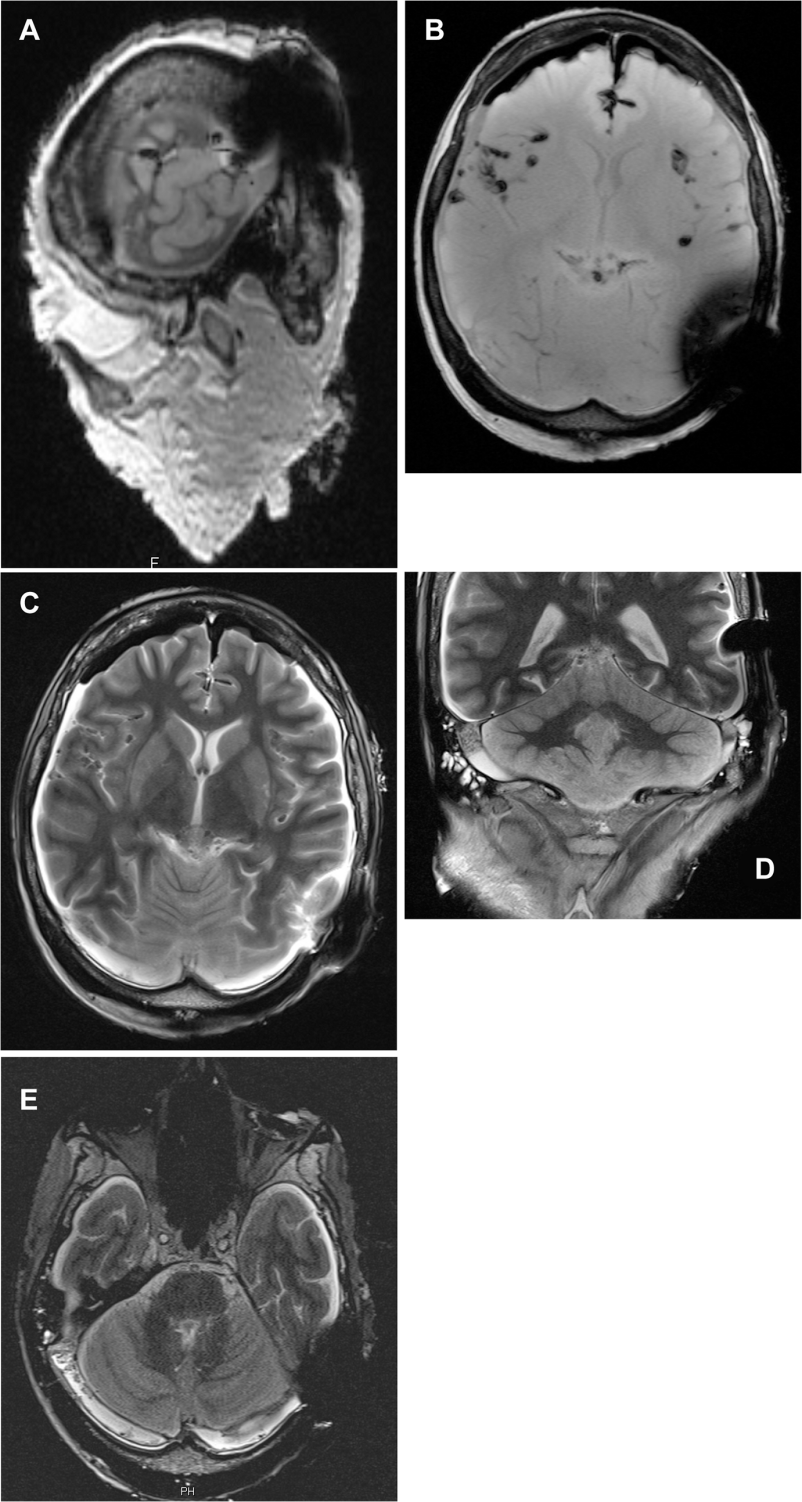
MR series of corpse head at 3T after magnet removal with non-magnetic spacer in situ. 5a: T1w MPR sagittal; 5b: T1w axial; 5c: T2w axial; 5d: T2w coronal; 5e: CISS. The MR images show the maximum diameter of the artefact in each sequence.
